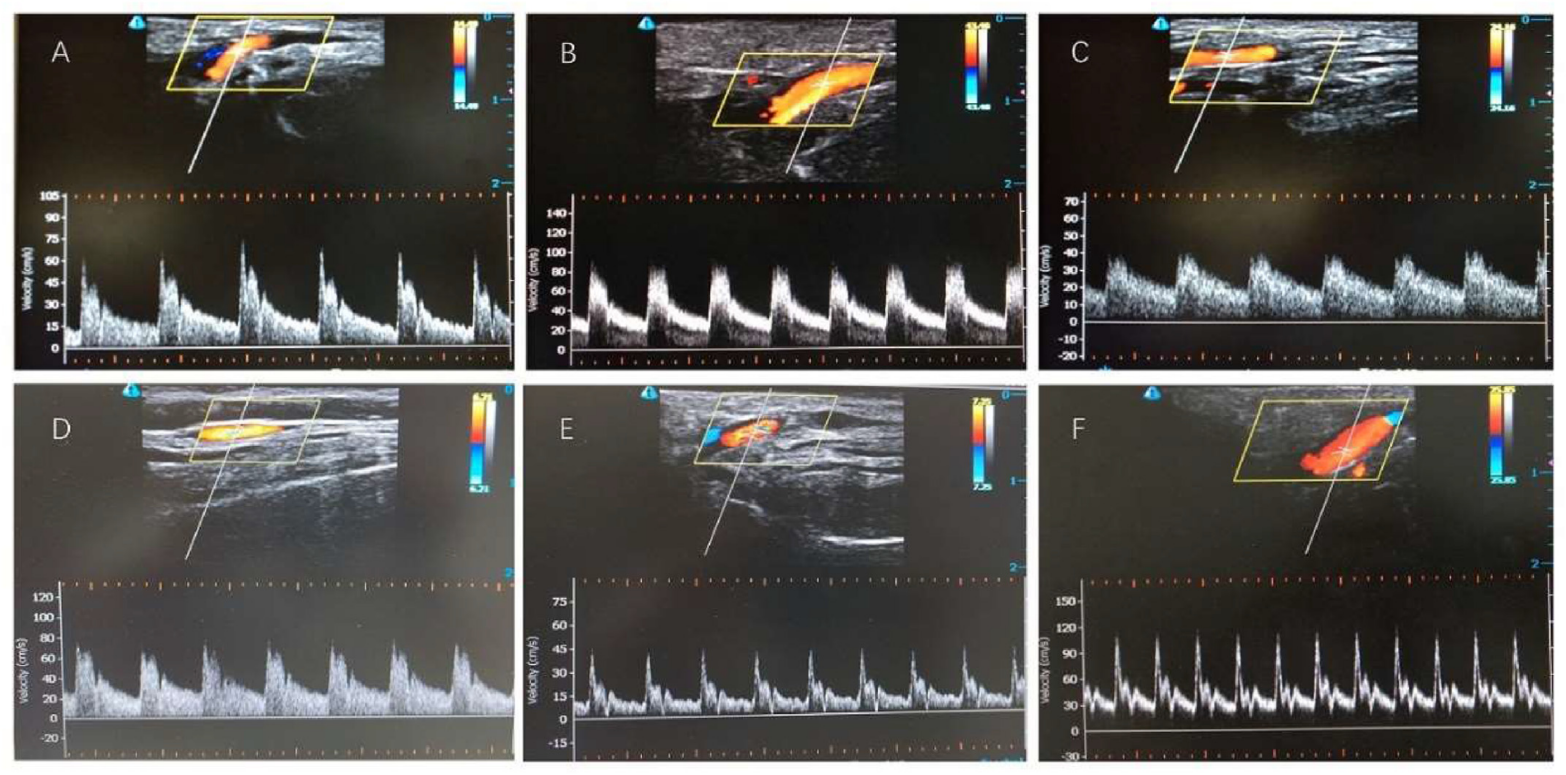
Color Doppler spectrogram of the STA blood flow between low and high resistant pattern. A. preoperative spectrogram of the low resistant pattern B. perioperative spectrogram of the low resistant pattern C. postoperative spectrogram in the follow-up of the low resistant pattern D. preoperative spectrogram of the high resistant pattern E. perioperative spectrogram of the high resistant pattern F. postoperative spectrogram in the follow-up of the high resistant pattern.
